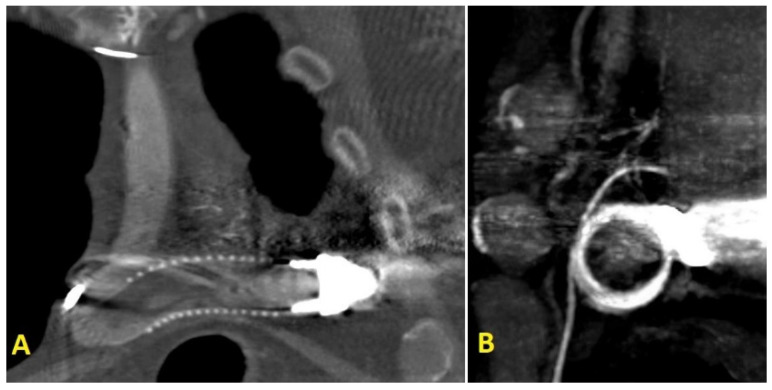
(A) chest CT-scan coronal-view focused on of LVAD outflow-graft; (B) CT-scan sagittal-view showed an image suggesting a thrombus in the proximal part of the LVAD outflow-graft.
